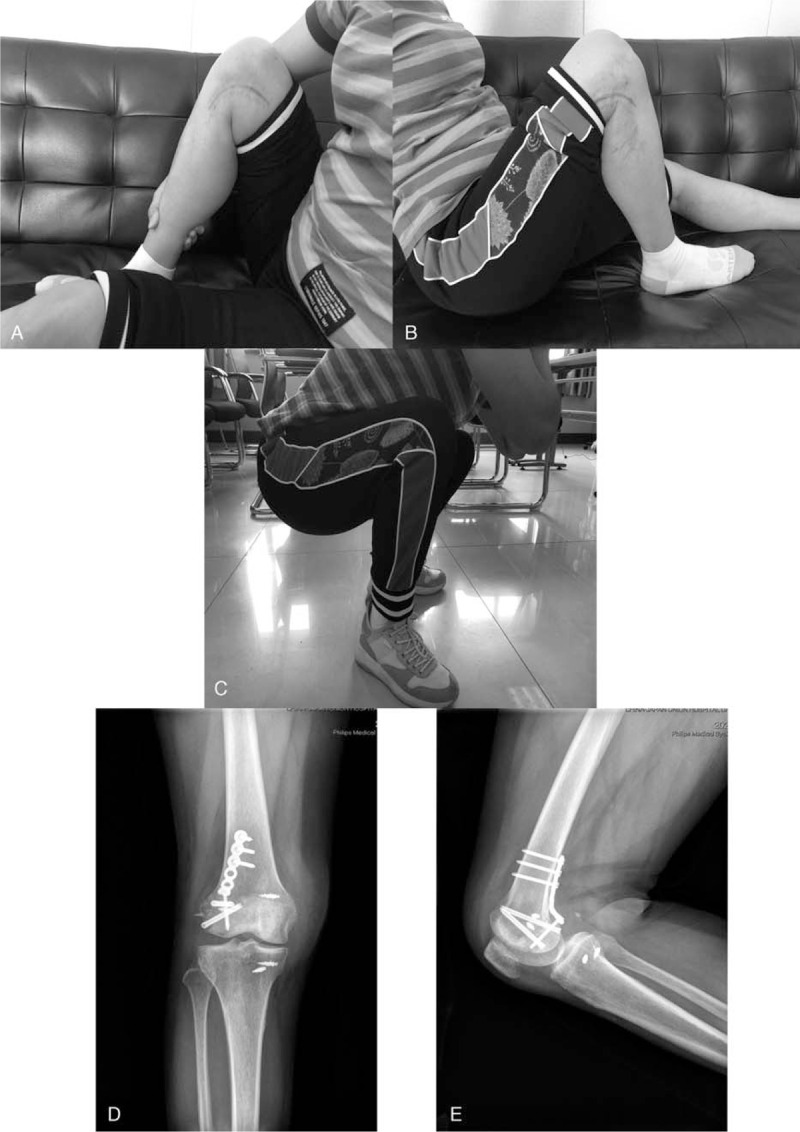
(A, B, C) After four months, the range of motion of the knee has achieved 0–130°. (D, E) AP view and lateral view showed the fracture line disappeared on the radiograph and achieved fracture healing.
